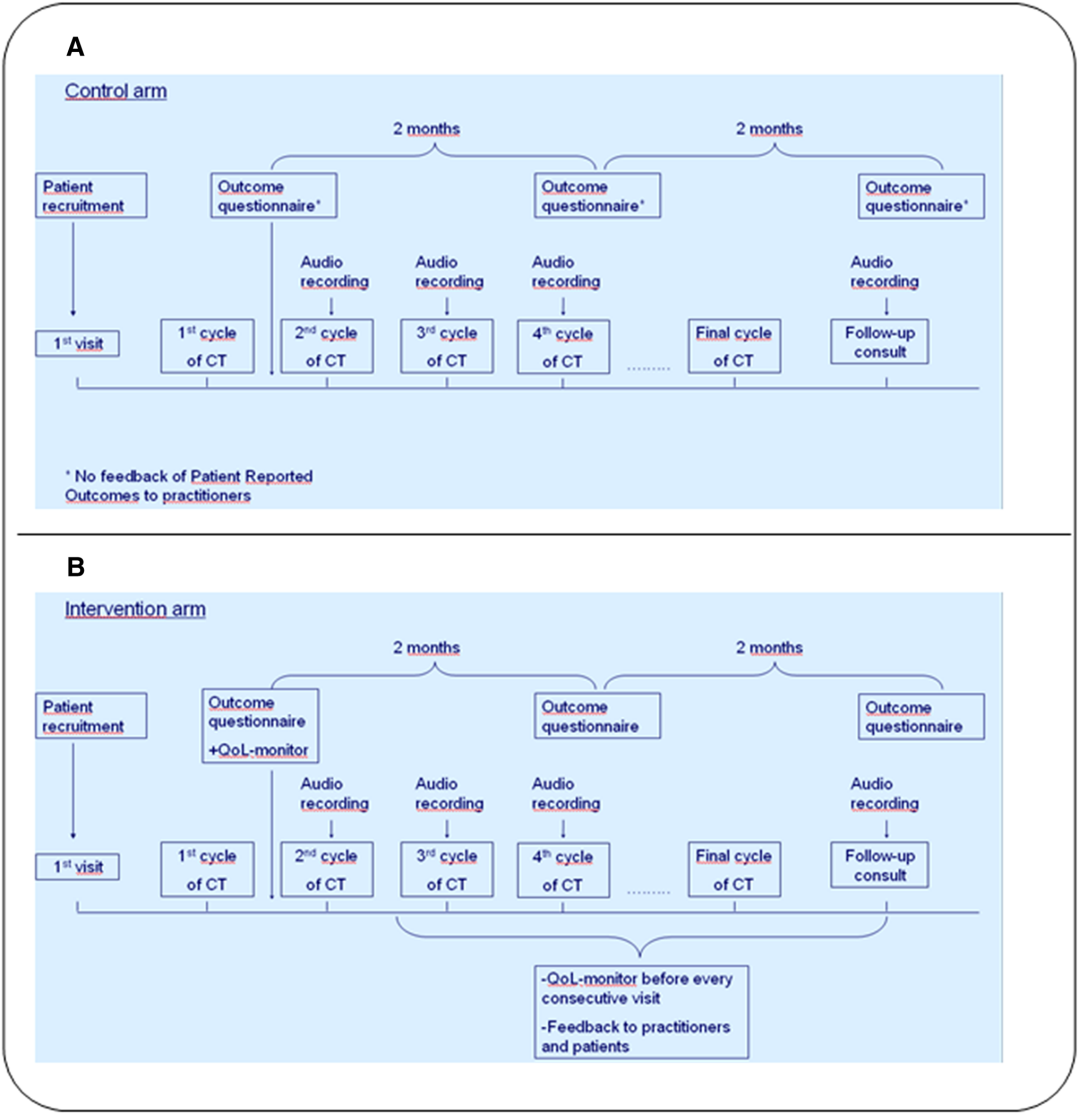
Timeline of assessments for patients in the control arm (a) and the experimental condition (b). The outcome questionnaire included assessment of QoL, illness perceptions, self-efficacy, satisfaction with communication and distress. The QoL-monitor consists of general and breast cancer specific QoL questionnaires, distress and care needs during and after treatment. *CT* chemotherapy
